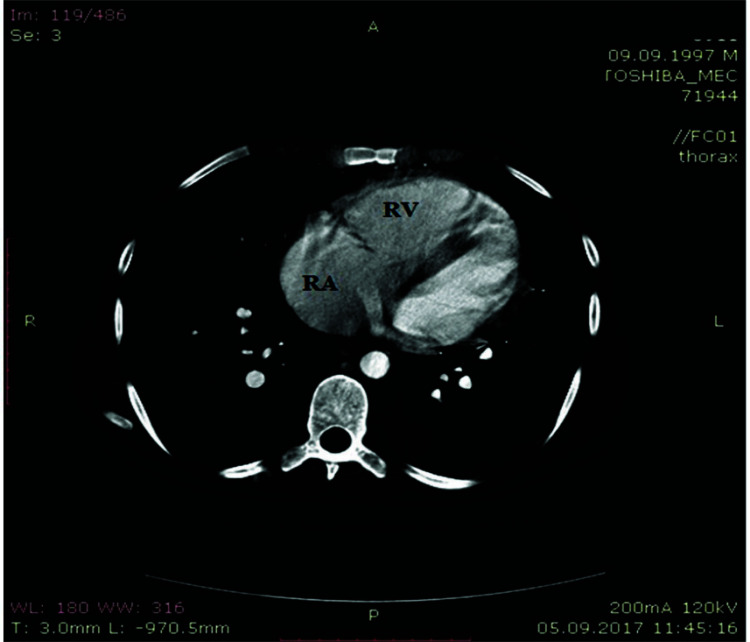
CT scan – the right heart enlargement.
